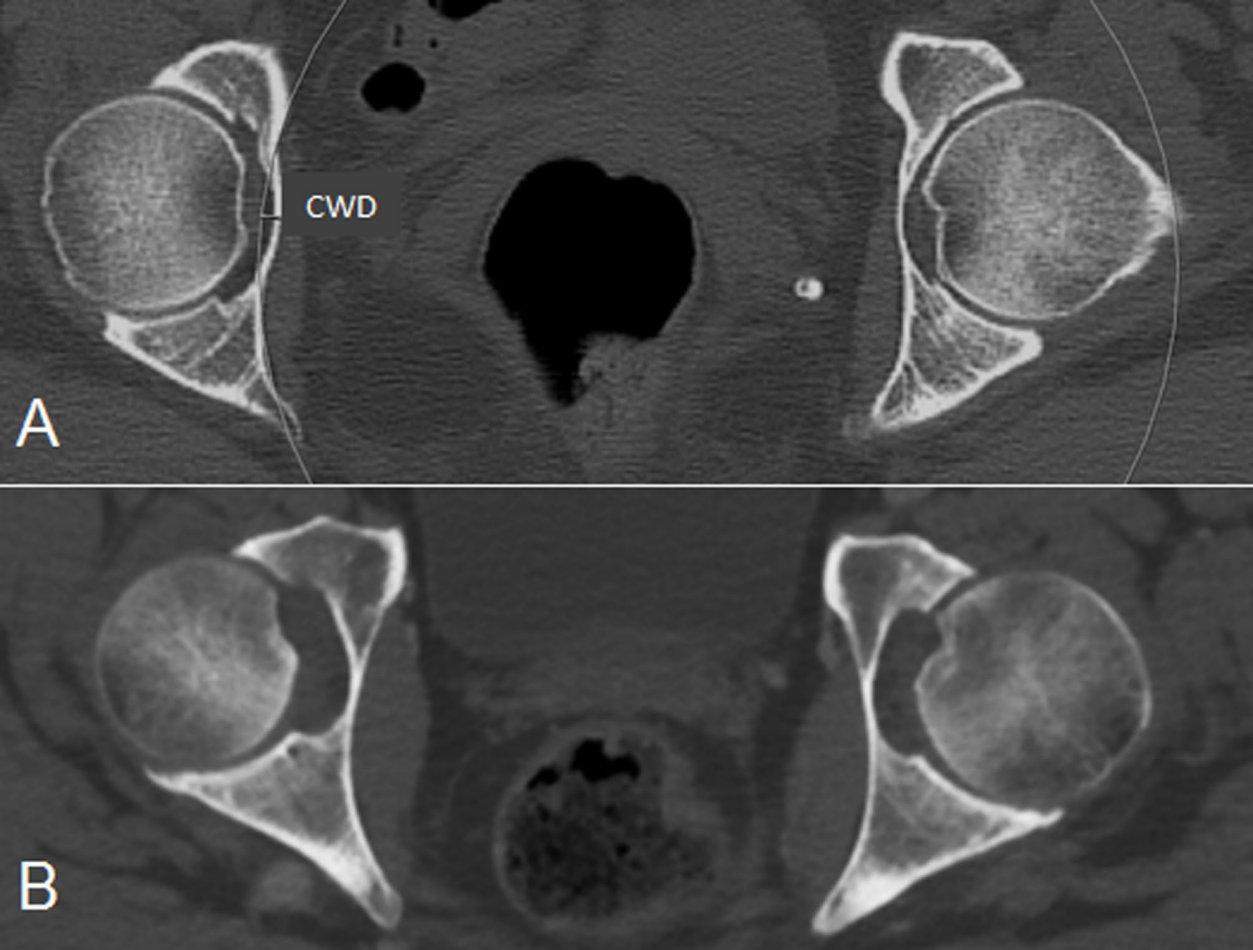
Axial CT images (3 mm thick sections) of the hips in two different patients. (A) Patient with Marfan syndrome and bilateral protrusio acetabuli. A circle with 10 cm radius was fitted to the inner pelvic wall of the right hip, and the degree of protrusion was measured as the distance between this circle and the most medial point of the inner pelvic wall of the acetabular fossa, the circle wall distance (CWD). (B) Normal hips for comparison
